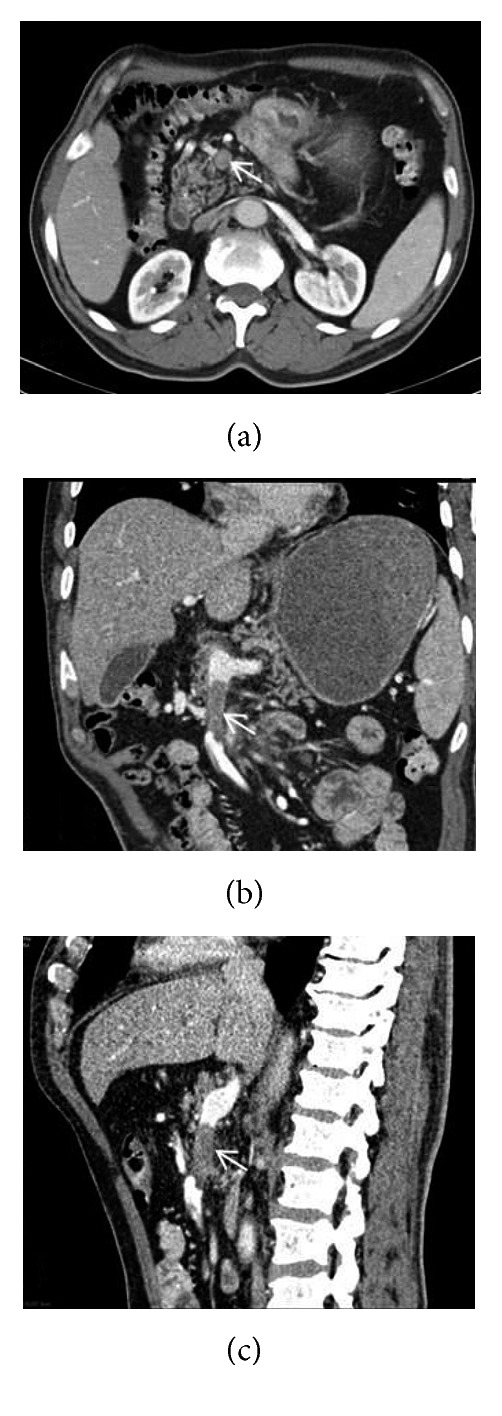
Axial (a), coronal (b), and sagittal (c) slices of abdominal CT show contrast media defect due to tumor thrombus (arrows) in the superior mesenteric vein.
